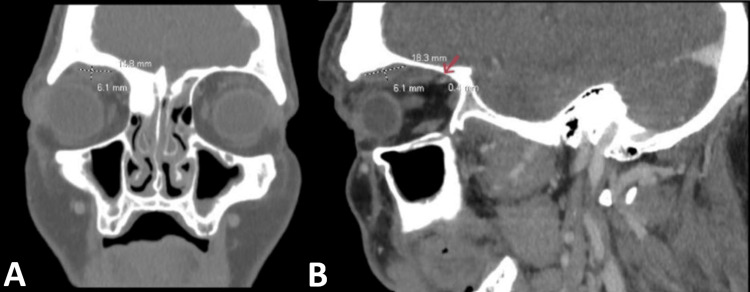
CT of the sinus with IV contrast CT of the sinus with IV contrast showed large ossified lesions compatible with an osteoma nearly completely occluding the right frontal sinus and extending into the left frontal sinus (A: coronal view; B: sagittal view). The subperiosteal abscess (marked by dash lines) in the right orbital wall measuring 1.83×1.48×0.6 cm with associated mild exophthalmos was stable compared to the previous CT of the orbits. The right frontal sinus subgaleal abscess/phlegmon (red arrow) has improved in thickness from 0.6 cm to 0.4 cm compared to the last CT of the orbits.
